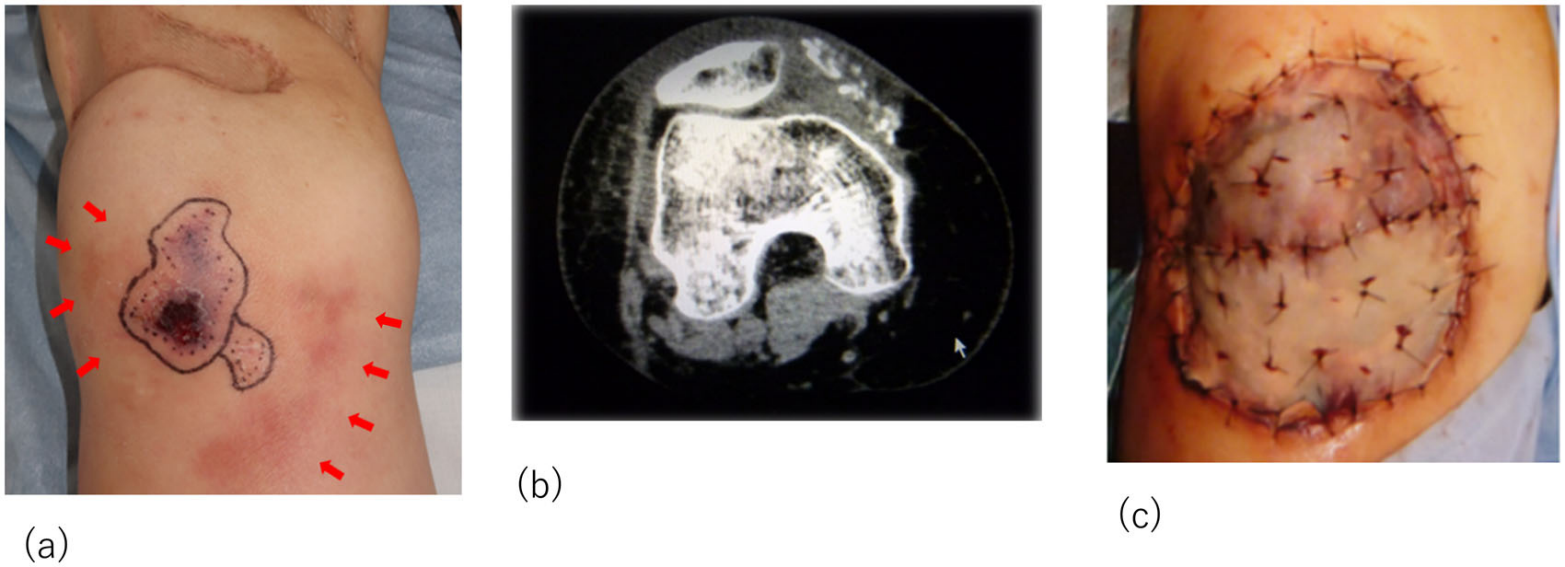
Recurrent lesion on the right knee. (a) The lesion on the right knee: obvious nodule with purpura and skin necrosis is marked. Arrows indicate the inflammation spreading around the knee. (b) Computed tomography images showing calcification and inflammation at the knee joint. (c) The skin graft was applied as a sheet in the knee joint area.
